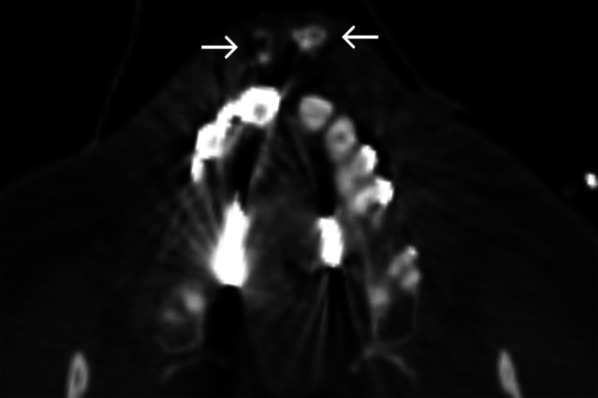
Broken teeth. Axial CT image showing multiple dislocated hyperdense objects in the upper lip (arrows)
